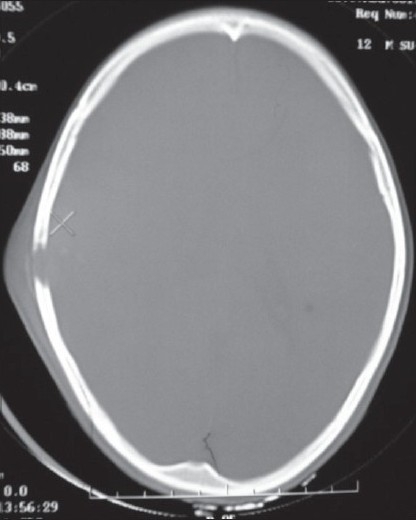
Plain CT-scan of head (bone window) showing lytic area in the right side of the parietal calvarium.
